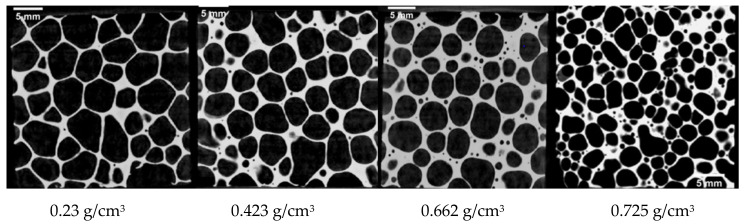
CT scan images of internal holes in the aluminum foam materials with different densities (thickness of 30 mm).
